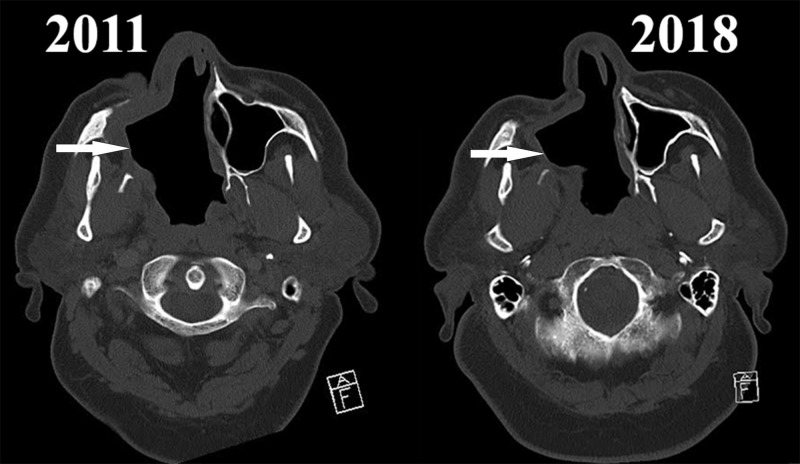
H&N CT scans from 2011 to 2018 – the neocavity (arrows) shows no significant changes. H&N: Head and neck; CT: Computed tomography
